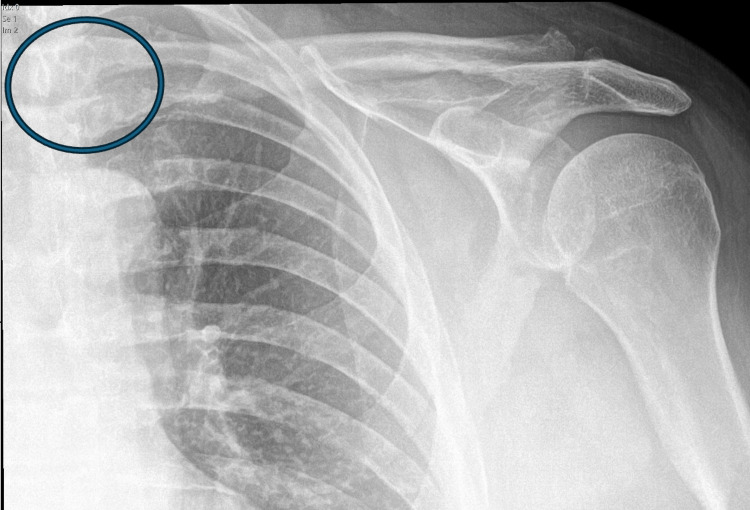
Postoperative anteroposterior radiograph of the left shoulder Anteroposterior radiograph of the left shoulder demonstrating resection of the medial (sternal) end of the clavicle (circled in blue). Joint alignment is preserved with no evidence of residual dislocation or acute fracture.
